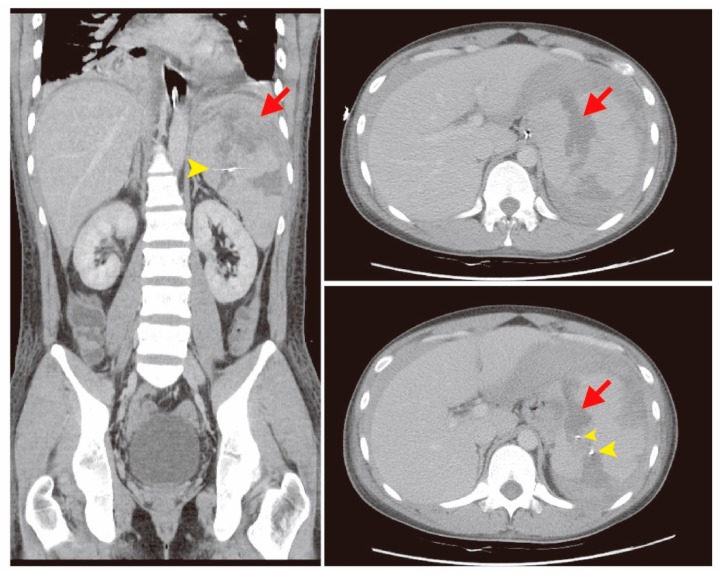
In the follow-up computed tomography (CT) scan, partial infarct of the spleen (red arrows) with two-coil embolization was noted, with improved hemoperitoneum (yellow arrow heads).
